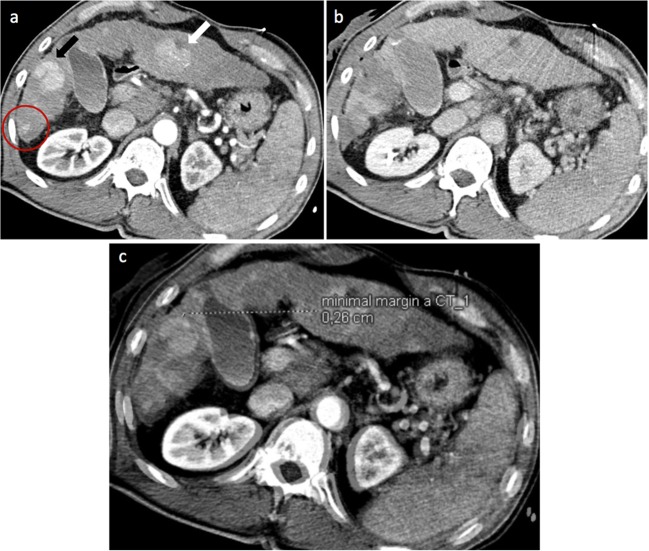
Registration failure of a lesion in a 58-year-old male. a The arterial phase of pre-SRFA CT scan showing lesions in liver segment III with 4 cm in diameter (white arrow), in liver segment V with 3.2 cm (black arrow), and in liver segment VI with 1.6 cm (red circle). b The late portal venous phase of post-SRFA CT scan with treated lesions in liver segments V and VI. The lesion in liver segment V was treated in a second session 2 months later. c The successful fusion of pre- and post-SRFA CT scan with the successful fusion of the lesion in liver segment V (MAM 2.6 mm in clock position 12–2 h). An adequate fusion for the lesion in liver segment VI was not possible due to its subcapsular location and extensive liver deformation
